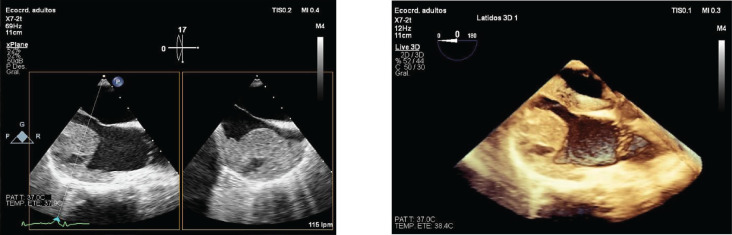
2D and 3D transesophageal echocardiography. Large mass protruding into the right atrium.
